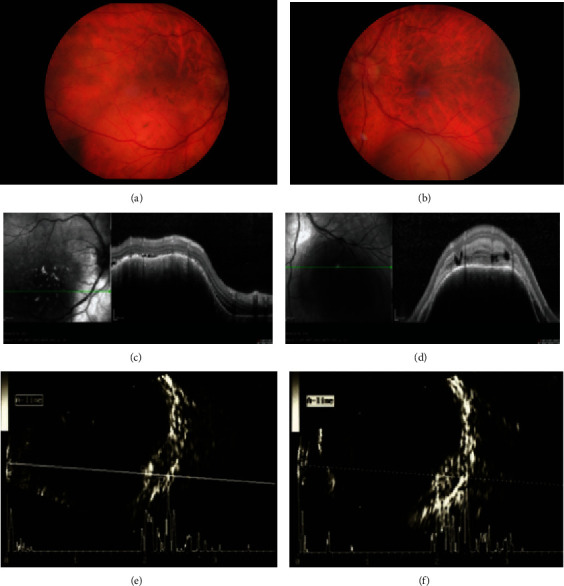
Color fundus image of the right eye (a) revealed an elevated, oval-shaped, lightly pigmented, orange, subretinal lesion in the inferotemporal quadrant with secondary serous retinal detachment extending to the macula. In the left eye (b), a nonpigmented, reddish-white, subretinal lesion below the inferior temporal arcade was observed. Examination with optical coherence tomography in the right eye (c) revealed eradication of the choriocapillaries and irregular choroidal surface overlying the lesion and in the left eye (d) showed a dome-shaped choroidal morphology, compression of the overlying choriocapillaris, and intraretinal fluid at the site of the lesion. Ultrasound examination showed dome-shaped choroidal lesions in the right (e) and left (f) eyes with a medium-to-high internal reflectivity and a dense acoustic sign.
